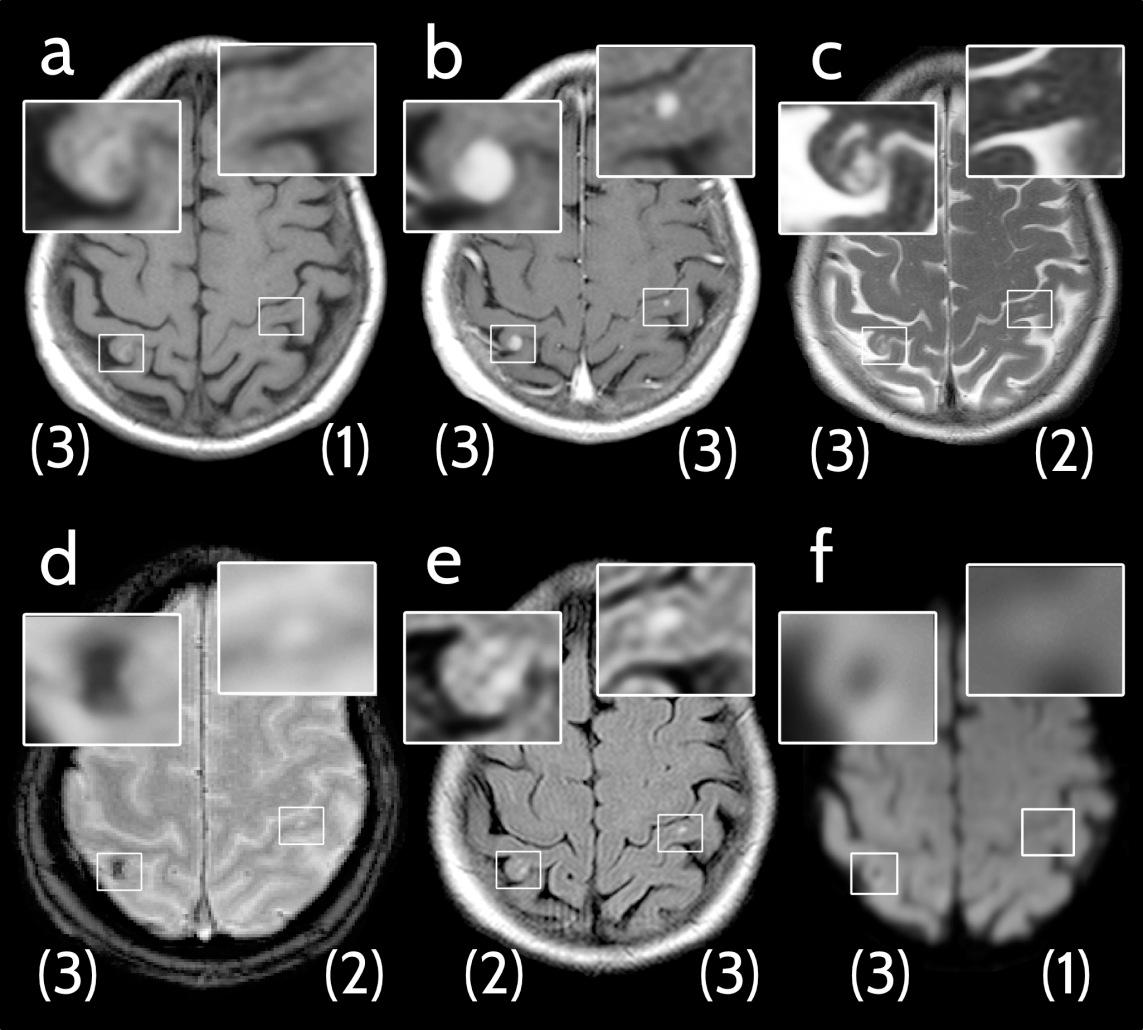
Example of applied image analysis. Axial cMRI scans of a 70-year-old woman with newly diagnosed brain metastases from malignant melanoma (MBM). Conspicuity scores of each MBM are indicated in brackets below the respective lesion. (a) Non-enhanced T1-weighted image, right MBM: conspicuity score (CS) 3, left MBM: CS 1. (b) Contrast-enhanced T1-weighted image, right MBM: CS 3, left MBM: CS 3. (c) T2-weighted image, right MBM: CS 3, left MBM: CS 2. (d) Fast low angle shot 2D susceptibility-weighted imaging (SWI), right MBM: CS 3, left MBM: CS 2. (e) Fluid-attenuated inversion recovery (FLAIR) image, right MBM: CS 2, left MBM: CS 3. (f) Diffusion-weighted image (DWI TRACE), right MBM: CS 3, left MBM: CS 1.
